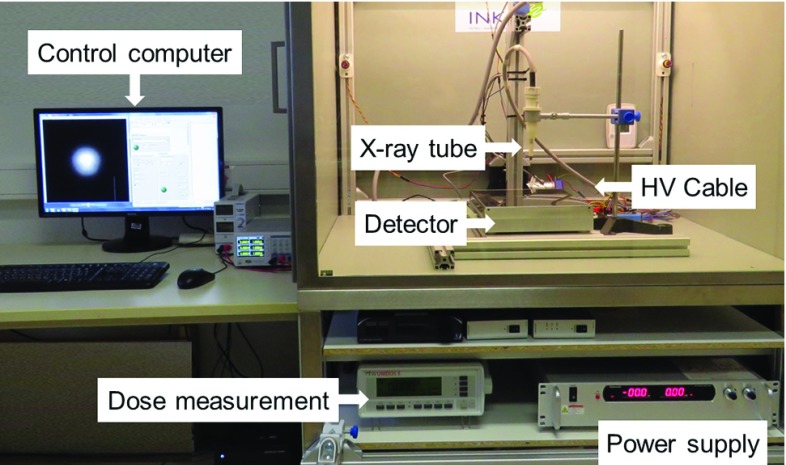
Shielded measure and test set-up for X-ray tubes in the INKA laboratory
